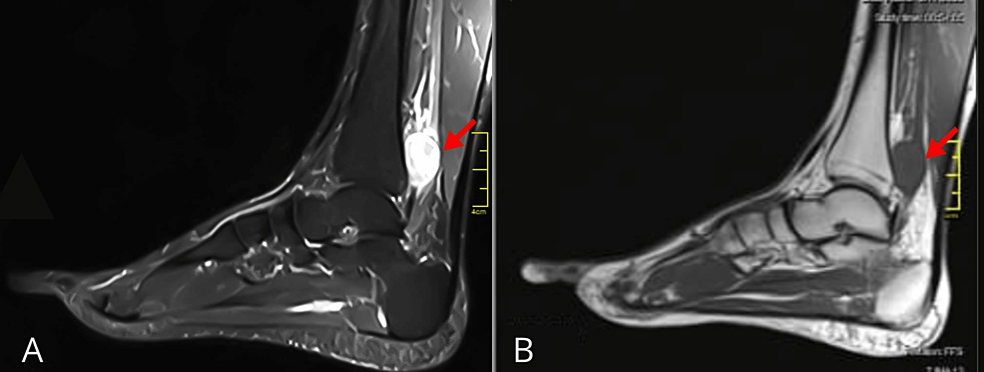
Magnetic resonance imaging (sagittal view) of the patient exhibiting heterogeneously increased signal intensity on T2-weighted image (A) while showing iso-intensity on T1-weighted image (B) of the foot (red arrow)
